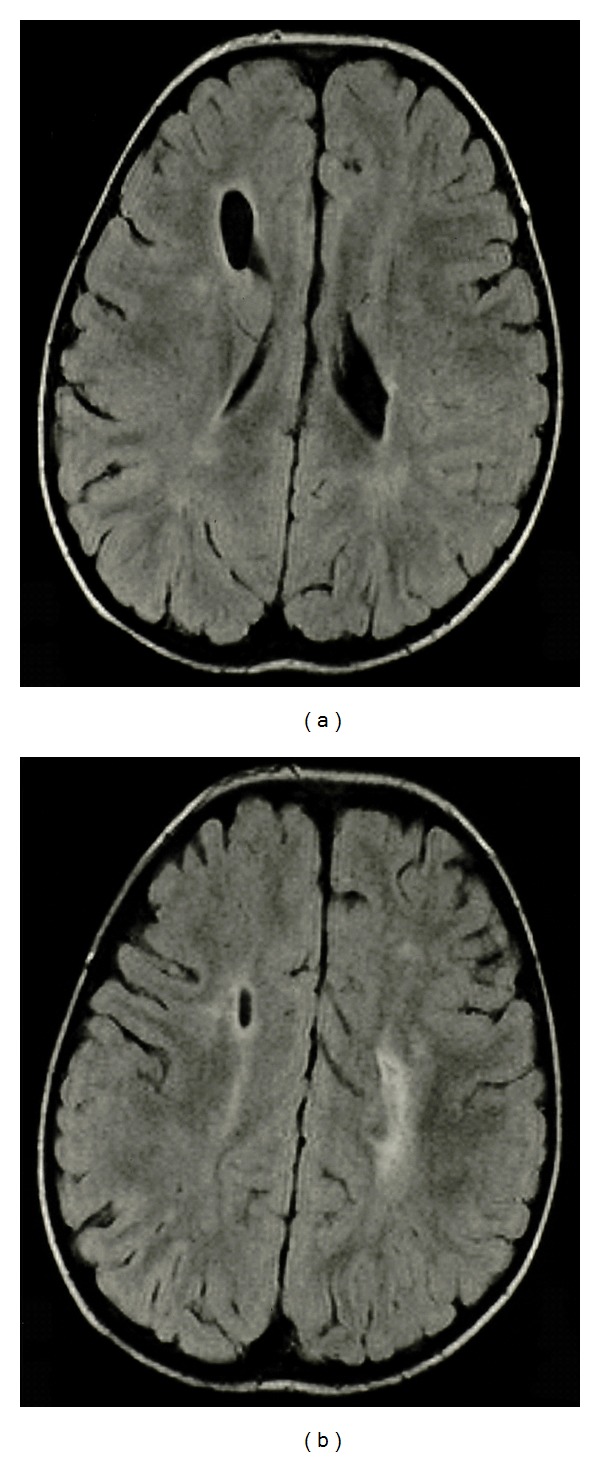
Transverse FLAIR MRI from the first case, family 2. Note irregular ventricular border in (a) and in a more rostral section, white matter hyperintense signal more prominent on the left in (b).
